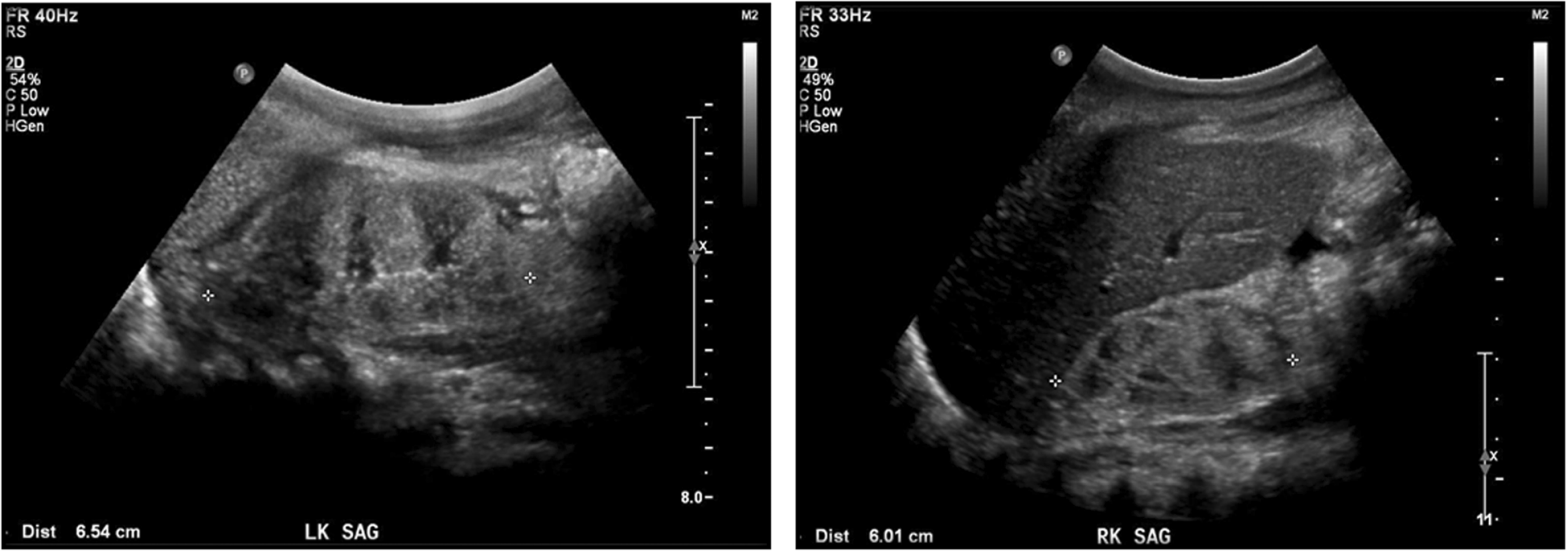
Renal ultrasound at presentation. The patient’s kidneys were notable for small size, diffuse echogenicity and loss of corticomedullary differentiation. Average kidney size for 5-year-old children is 8 cm, however she was small for age, and for a 90 cm tall child the average kidney size is 7.1 cm with 95% prediction limits of 5.8 to 8.3 cm. The ultrasound was performed at the time she was anuric, hence the renal pelvis appears to be collapsed
